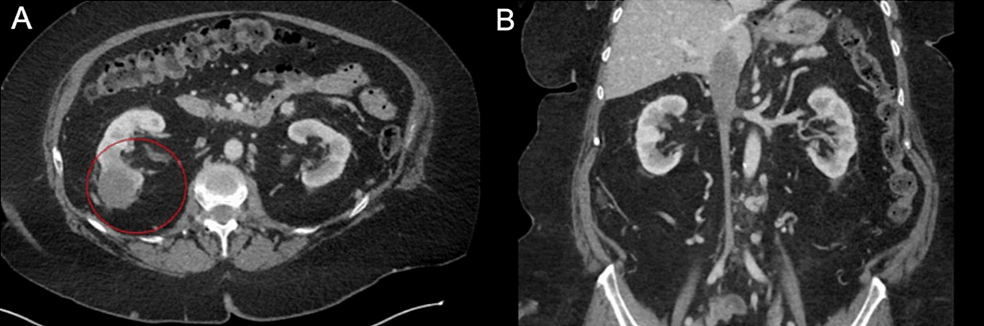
CT imaging of the abdomen and pelvis after one year of systemic therapy. (A) Axial view with significant necrotic shrinkage of primary renal mass. (B) Coronal view demonstrating necrotic shrinkage of thrombus below the diaphragm.
